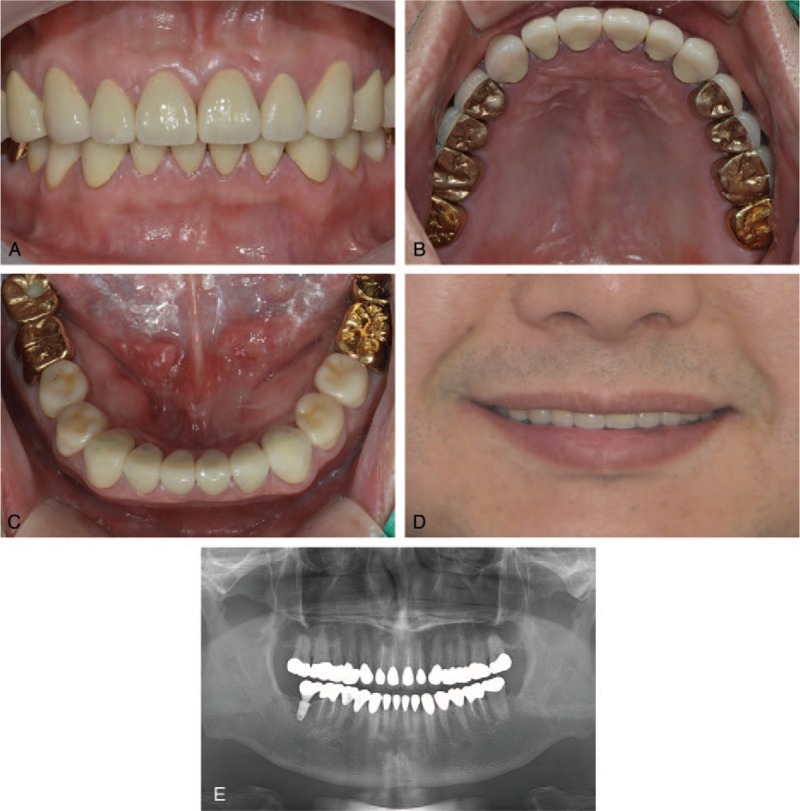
Contemporary combination of conventional and digital full-mouth restorations and postoperative situation. (A–C) Cast gold crowns on maxillary second molar and mandibular first and second molars. Porcelain-fused-to-gold crowns with gold occlusal surface on maxillary first molar and maxillary premolars. Monolithic zirconia crowns on mandibular premolars. Porcelain-fused to-gold crowns or porcelain-fused-to-zirconia crowns on maxillary and mandibular anterior teeth. (D) The resulting smile is similar to the presented digital smile design plan. (E) Panoramic radiograph acquired at the 2-year follow-up shows good treatment outcome.
